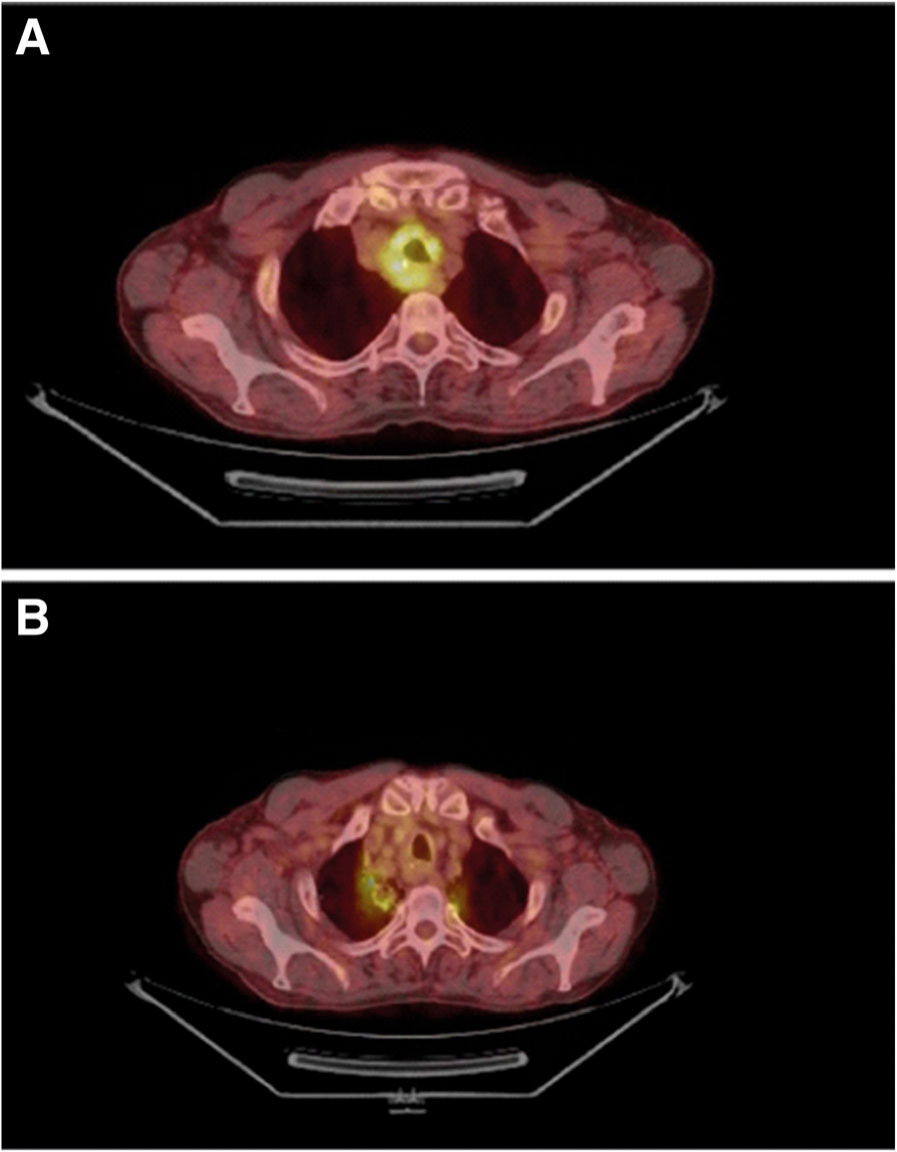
a, b Positron emission tomography-computed tomography before (a) and after (b) radiotherapy. The increased uptake of ^18^F-fluorodeoxyglucose at the level of trachea is not visible after the treatment. The increased uptake of ^18^F-fluorodeoxyglucose in correspondence with the superior pulmonary lobes refer to the local inflammation caused by radiotherapy
